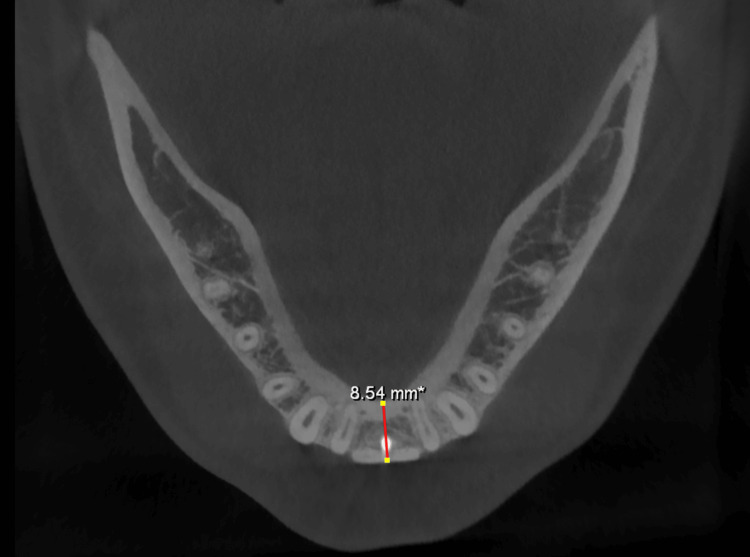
Postoperative CBCT (six months later) - axial view showing a mandibular ridge width of 8.54 mm. CBCT, cone-beam computed tomography
